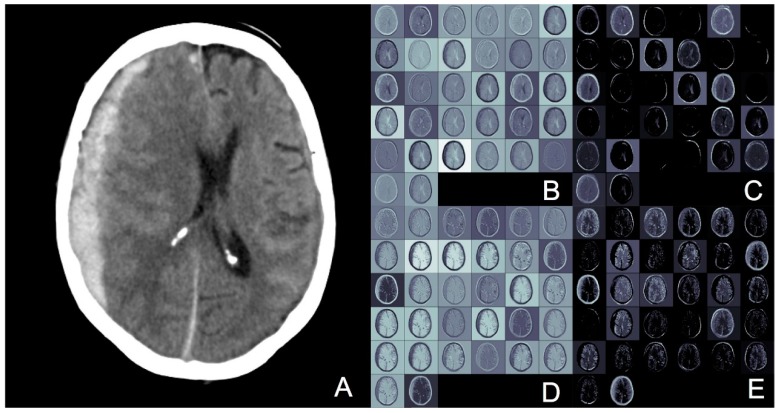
To demonstrate the effect of thresholding, a single slice of an ASDH CT Brain scan is shown, with the corresponding activations of convolution and pooling layers. A, original image. B, 1st convolution layer. C, 1st pooling layer. D, 1st convolution layer with thresholding applied. E, 1st pooling layer with thresholding applied. D and E appear sharper than B and C, demonstrating how thresholding can accentuate abnormal areas, and improve classifier performance.
